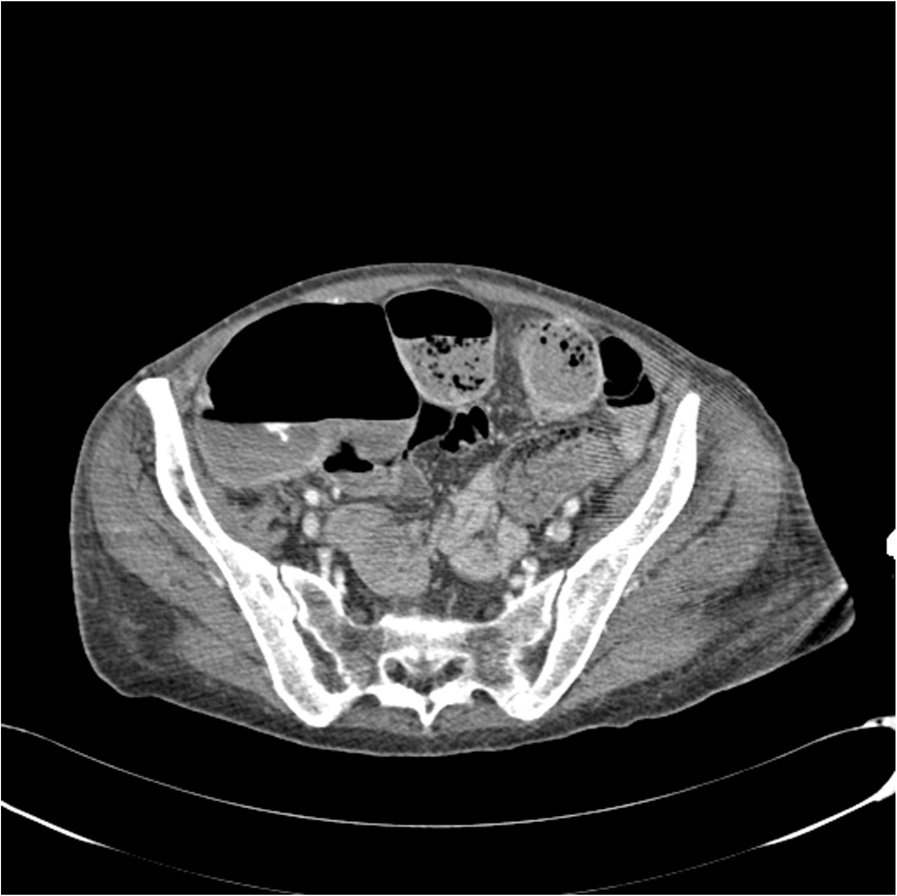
Axial CT-angiography image shows active arterial bleeding in the ascending colon
